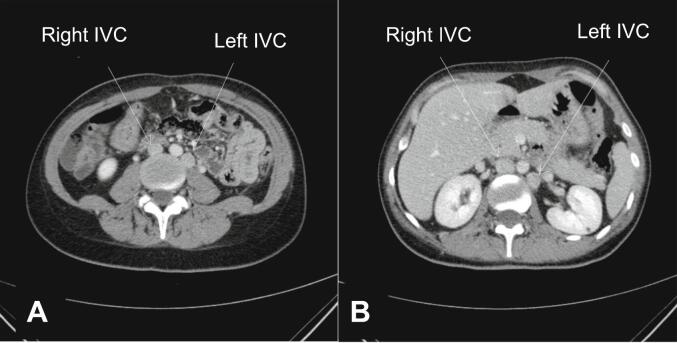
Axial images of contrast enhanced CT (portal phase) shows duplicated IVC at infra-renal (A) and renal levels (B).
